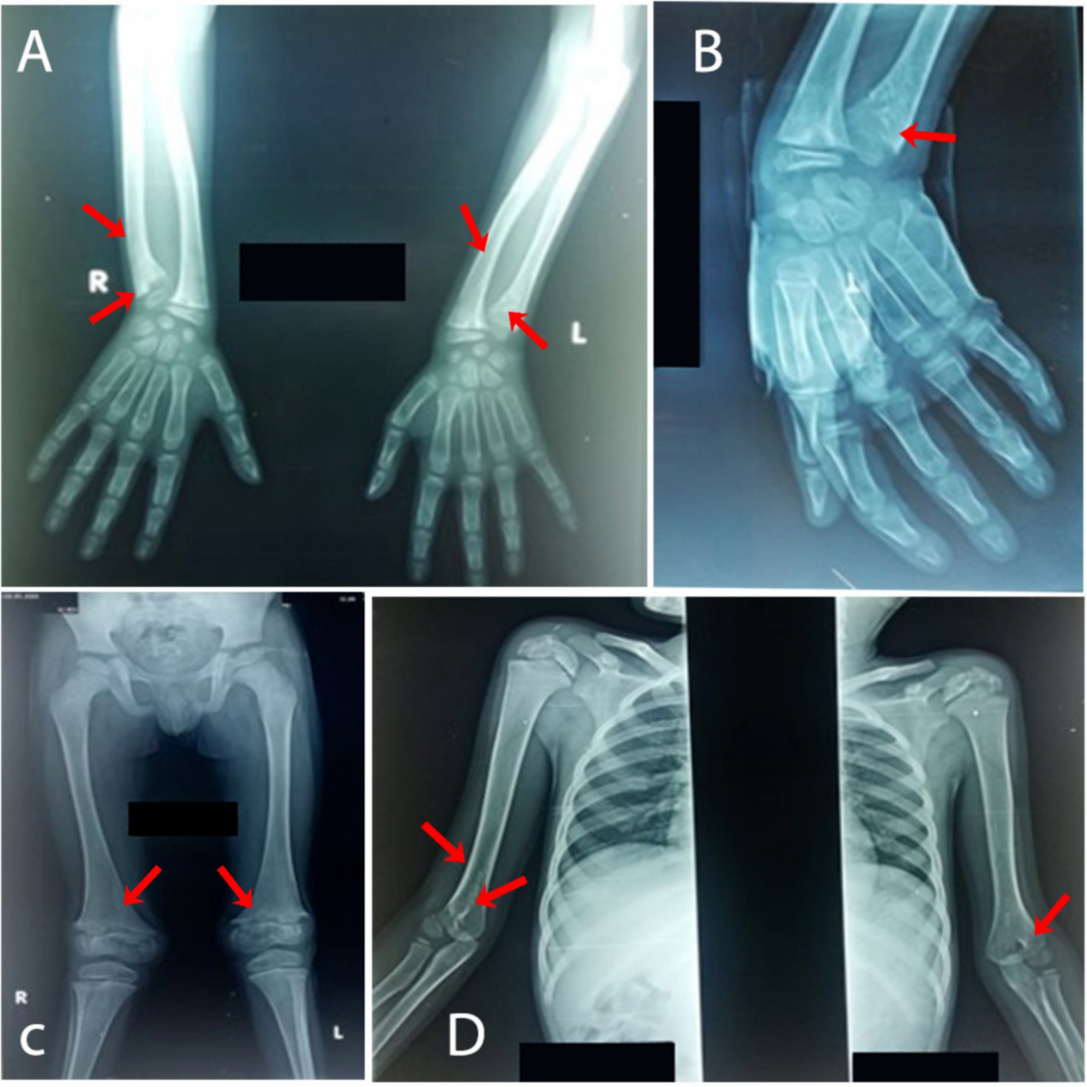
Conventional radiographs of the patient at eight years of age (A, B) X-ray of wrist joints showing irregularity and flaring at distal metaphysis of ulna bilaterally with bowing deformity; however, no fracture seen. (C) X-ray of pelvis and both femora showing irregularity at distal metaphysis of both femora without any fracture. (D) X-ray of both shoulders including humeri showing irregularity and flaring at proximal and distal humeral metaphysis with epiphyseal stippling.
